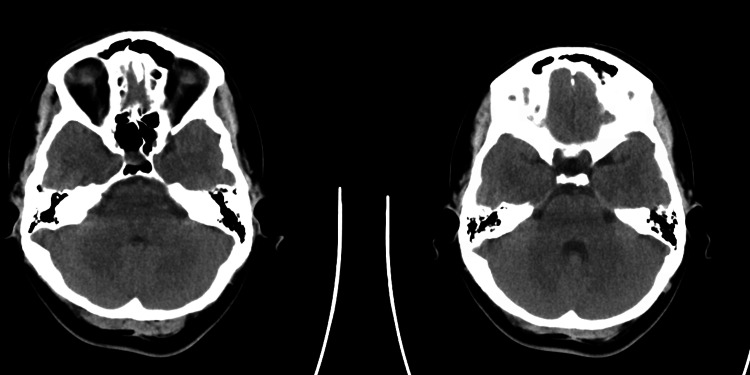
Essentially normal CT perfusion scan of the brain showing normal cerebral blood flow
